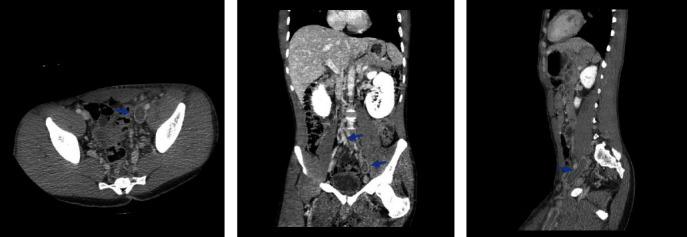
(a, b, c) CT abdomen/pelvis with contrast significant for occlusive thrombus in visualized portions of deep femoral veins, extending into the left common femoral vein, and into the left external, internal, and common iliac veins.
